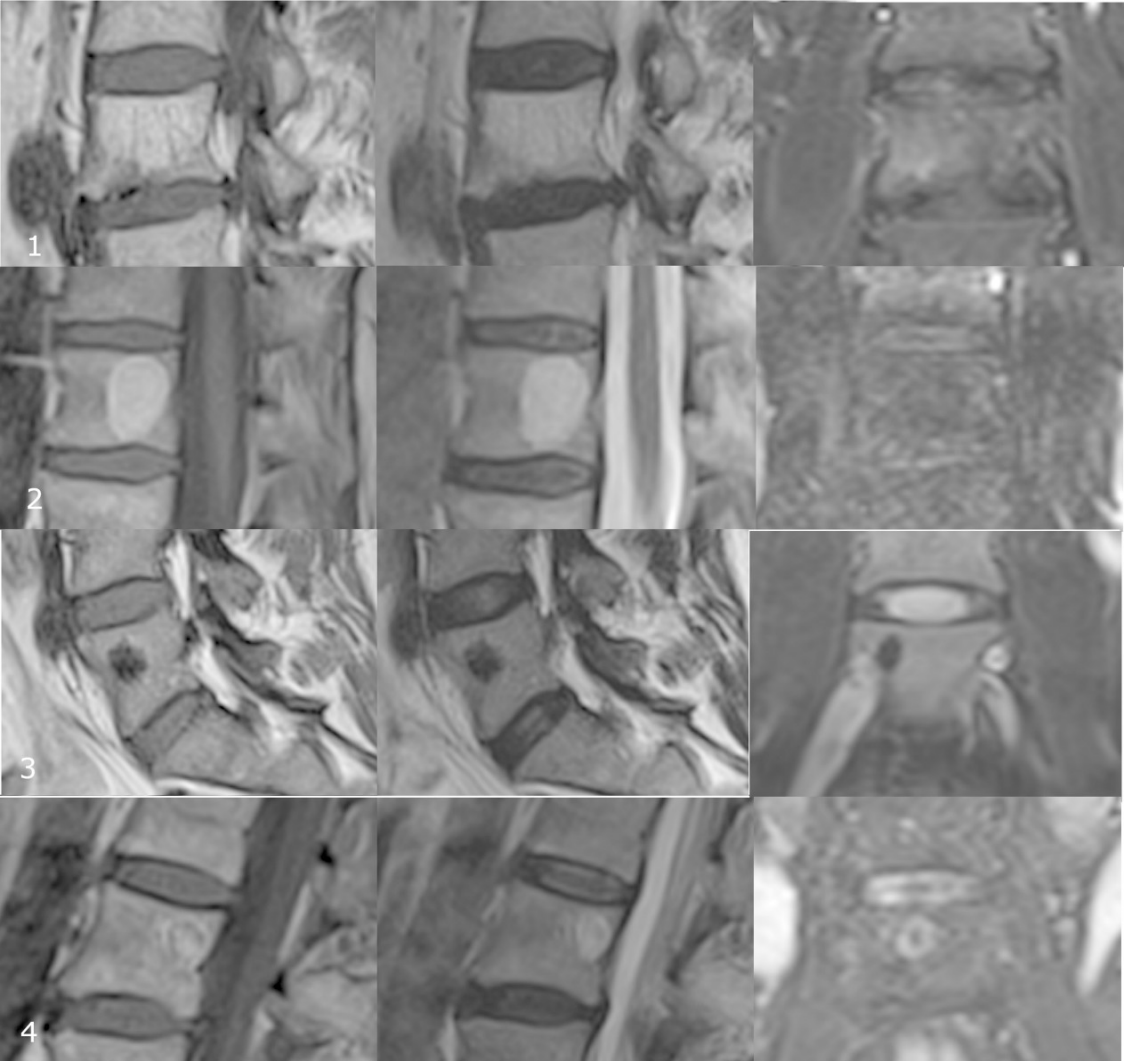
(1.) Examples for osseous lesions in MRI weighting of the vertrebra: 68Y old female with a hemangioma in a lumbar spine vertebrae 4. The osseus lesion is hyperintense in T1- and T2-weighted images and hyperintese as well as isointense in T2-weighted images with fat suppression and suggested as a fat-containing hemangioma with a typical striated pattern.; (2.) 74Y old female with a lipoma in thoracic spine vertebrae 12. The osseus lesion is oval, homogenous with hyperintense signal intensity in T1- and T2-weighted images and hypointense in T2-weighted images with fat suppression and suggested as an intraosseus lipoma.; (3.) 77Y old male with a sklerosis in thoracic spine vertebrae 5. The osseus lesion has a hypointense signal intensity in T1- and T2-weighted images as well as the T2-weighted images after fat suppression and suggested as a sklerotic osseus lesion or enostoma like in this case.; (4.) 68Y old female with a osteoblastoma in lumbar spine vertebrae 2. The osseus lesion has a low up to intermediate signal intensity on T1-weighted images with a low to predominantly high signal intensity in T2-weighted images without fat supression. In T2-weighted images with fat supression the osseus lesion reveals a hyperintense rim and a central hypointensity which could be interpreted as a nidus. This lesion might be suggested as an ostoidostoma and was categorized as an osteoblastom.
